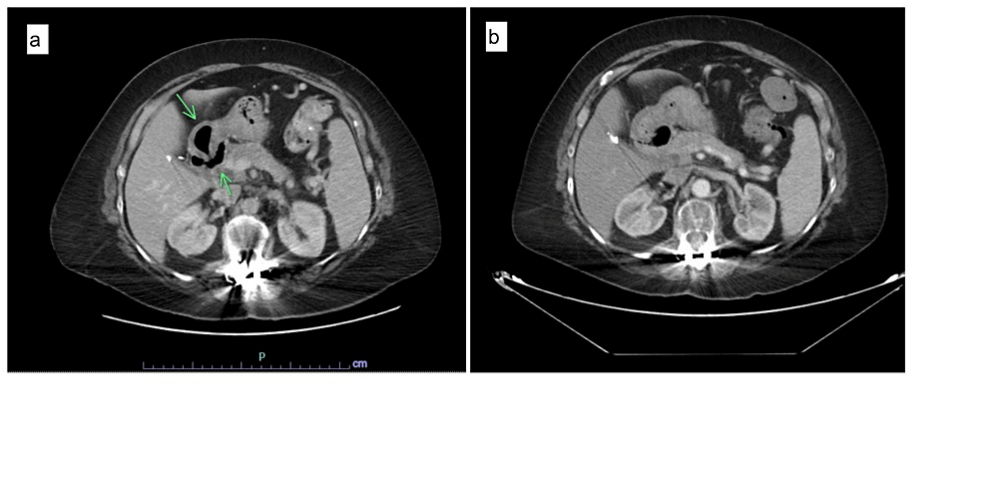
CT abdomen and pelvis demonstrate apple core lesion involving hepatic flexure of the colon (a). Normal hepatic flexure of the colon in previous CT abdomen for comparison (b).
